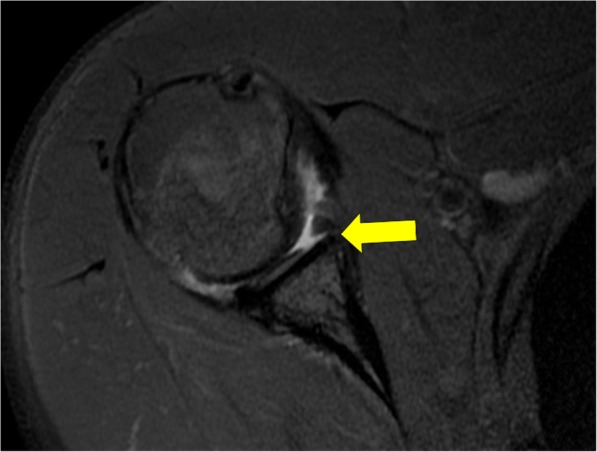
Female rugby player in her late 20’s: Axial T2-weighted fat-suppressed MRI demonstrates tear of the anterior inferior labrum (Bankart) lesion (arrow) and adjacent high grade chondral loss over the glenoid
